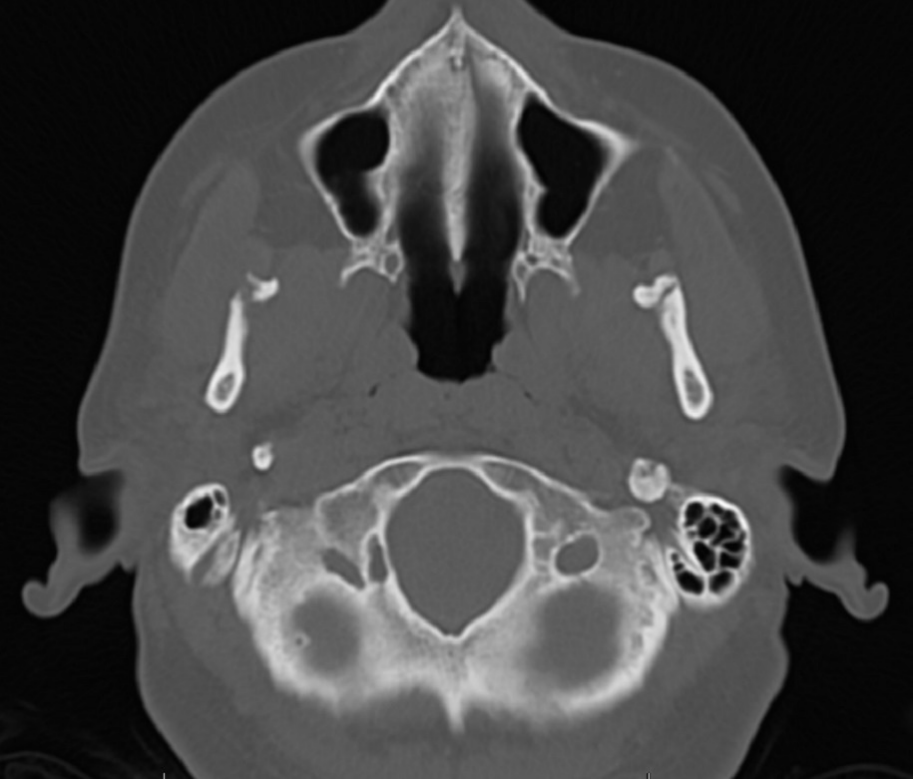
Axial CT shows isolated bilateral coronoid process fracture
